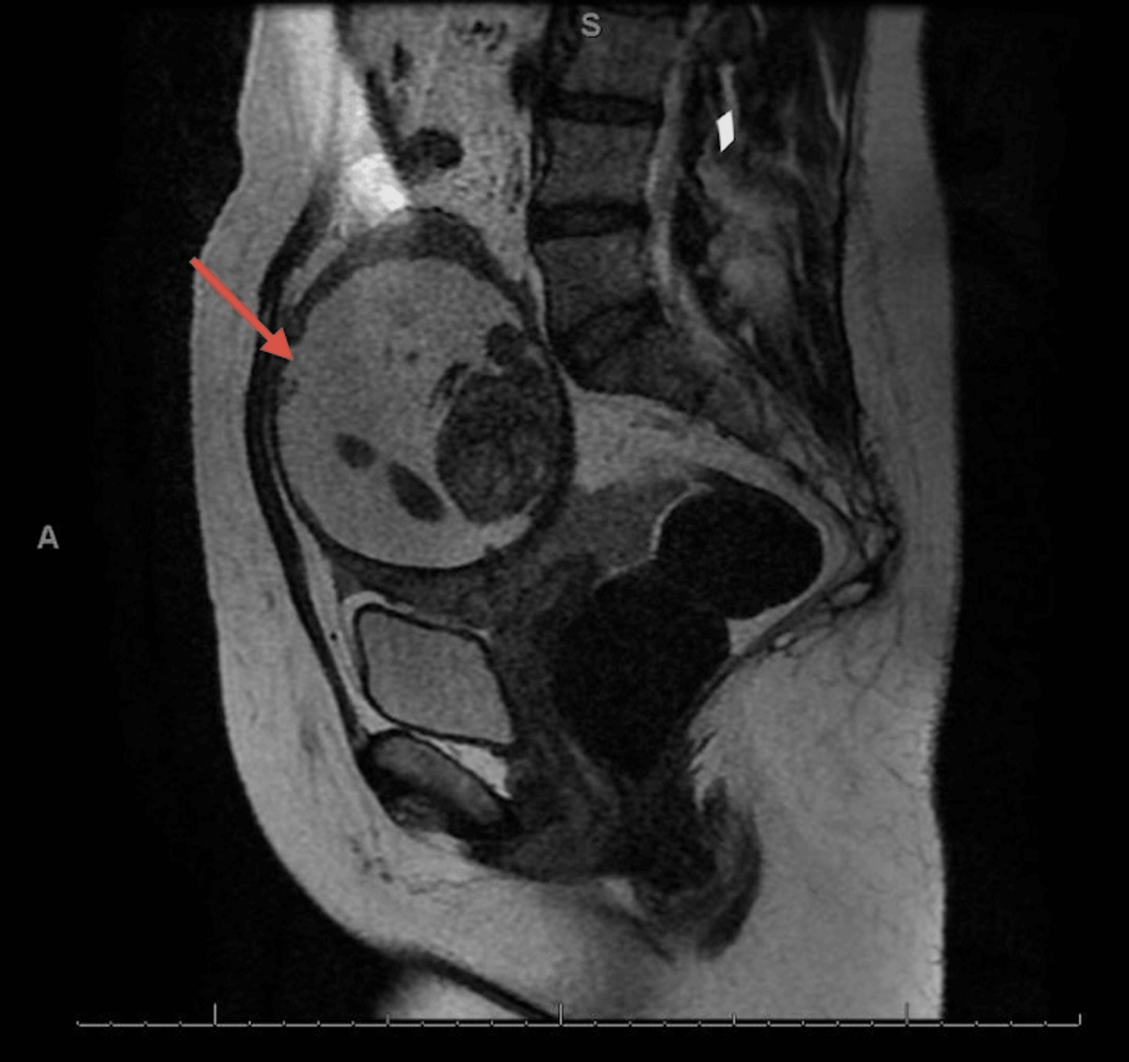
Sagittal image of MRI showing rudimentary horn pregnancy (red arrow).
